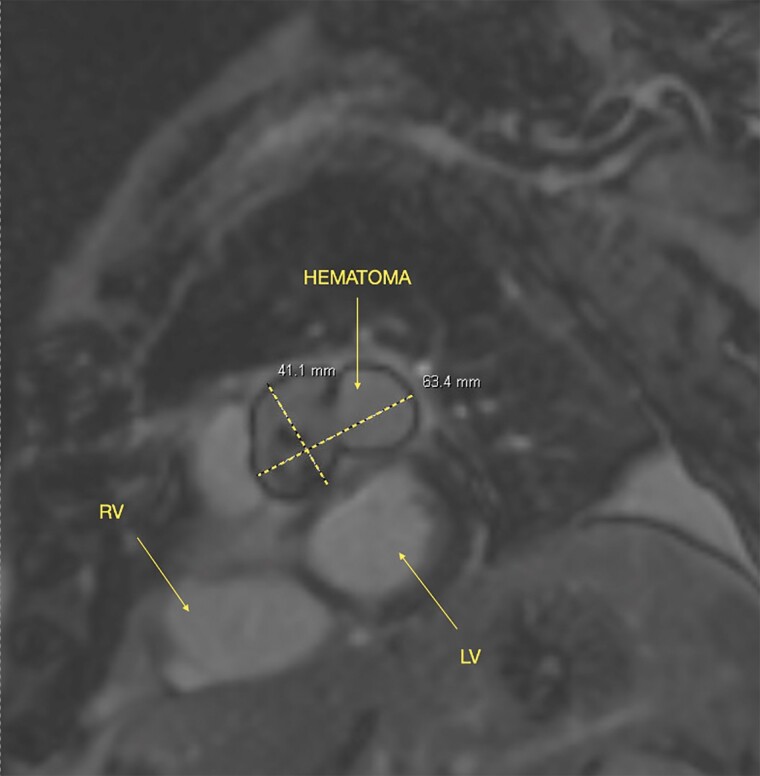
Cardiac MRI. Hematoma, haematoma; RV, right ventricle; LV, left ventricle.
